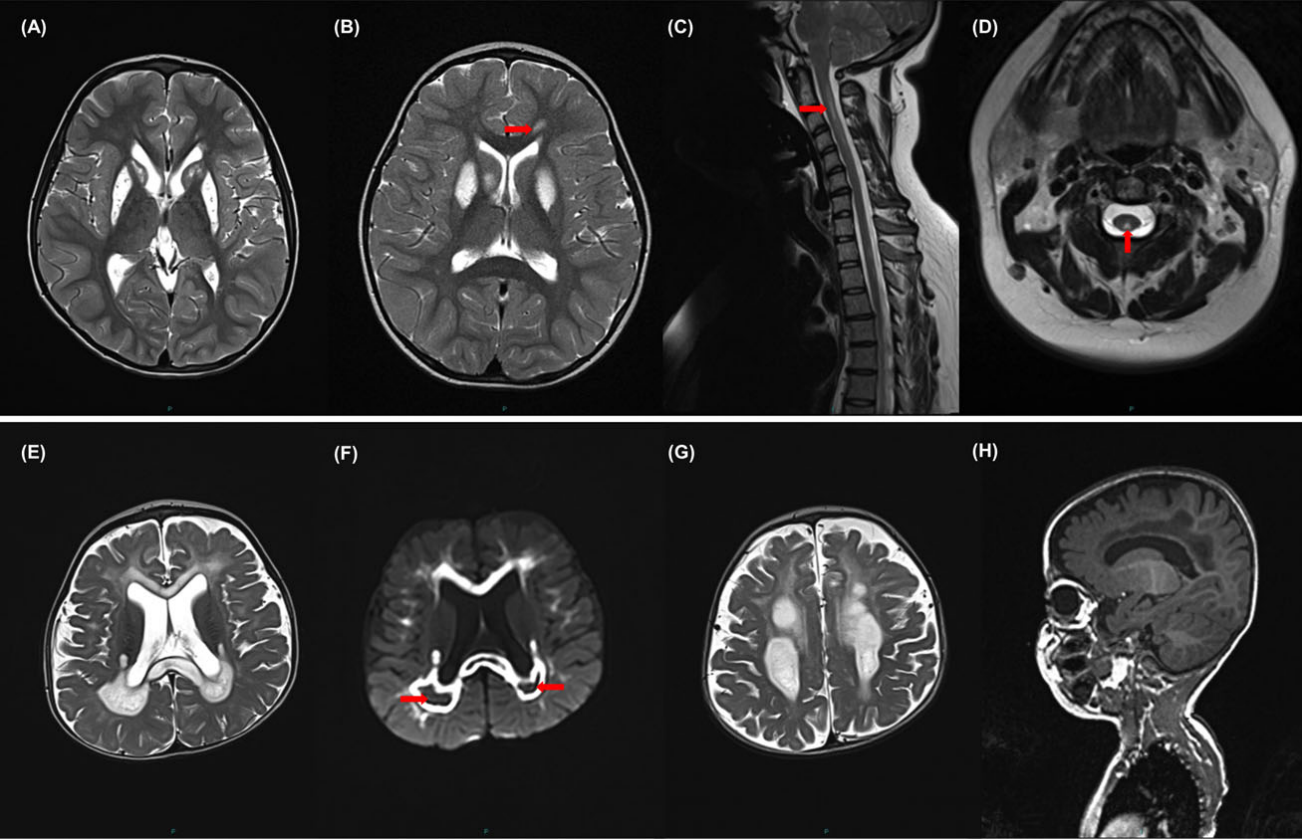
MRI head and cervical cord. T2‐weighted axial views show symmetrical hyperintensities in the striatum (A) (P4), hyperintensities in bilateral putamen, right caudate nucleus and a small white matter change in the left genu of corpus callosum (arrow) (B) (P2). T2‐weighted sagittal view shows an intrinsic T2 hyperintensity spanning C1‐4 level (C), with a corresponding change identified on the axial view (D) (P3). Extensive T2‐hyperintensities with areas of cavitation seen in the genu and splenium of the corpus callosum (E) and the posterior periventricular white matter with restricted diffusion, but not within the areas of cavitation (arrows) (F). (G) Large cystic changes with surrounding hyperintense T2 signal abnormality are present in the deep white matter. T1‐weighted sagittal view shows the confluent signal abnormality of the corpus callosum (H) (P1).
